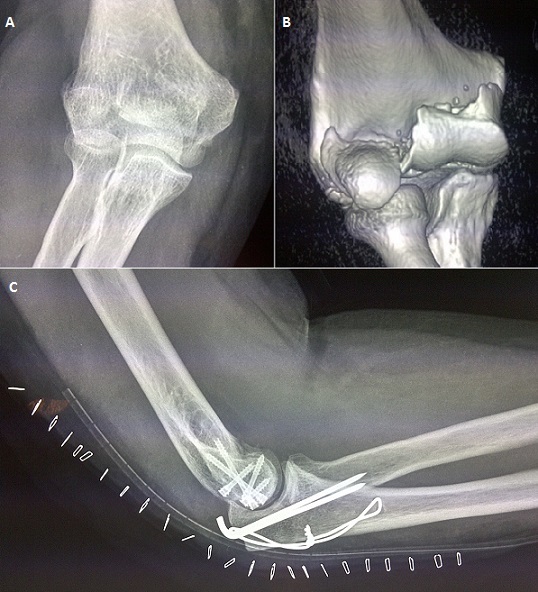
A) radiographie du coudemontrant la fracture frontaleemportanttoute la surface articulaire; B) reconstruction en 3D des images scanographiquesmontrant le trait de fracture frontal. Un deuxième trait scinde la surface articulaire en deux, condyle ettrochlée; C)radiographie de contrôle, abord par ostéotomie de l'olécraneetostéosynthèse par vissage
